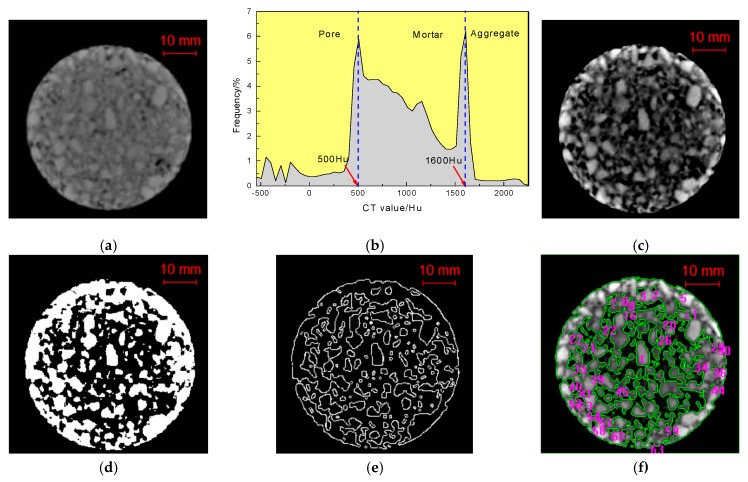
Digital image processing technology for the crack extraction procedure. (a) CT gray image, (b) CT number distribution, (c) Enhanced image, (d) Binary image, (e) Skeleton on binary image, (f) “AOI” selection.
